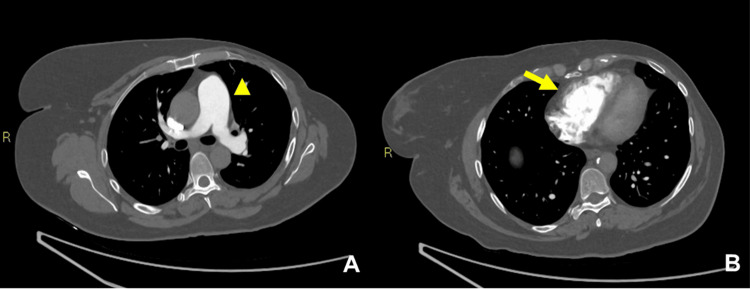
CT pulmonary angiogram to exclude pulmonary embolism CT pulmonary angiogram showing the dilated main pulmonary artery (yellow arrowhead, Panel A) with no pulmonary emboli in the proximal pulmonary artery branches, and the dilated right ventricle (yellow arrow, Panel B). Left mastectomy with absence of left breast tissue
